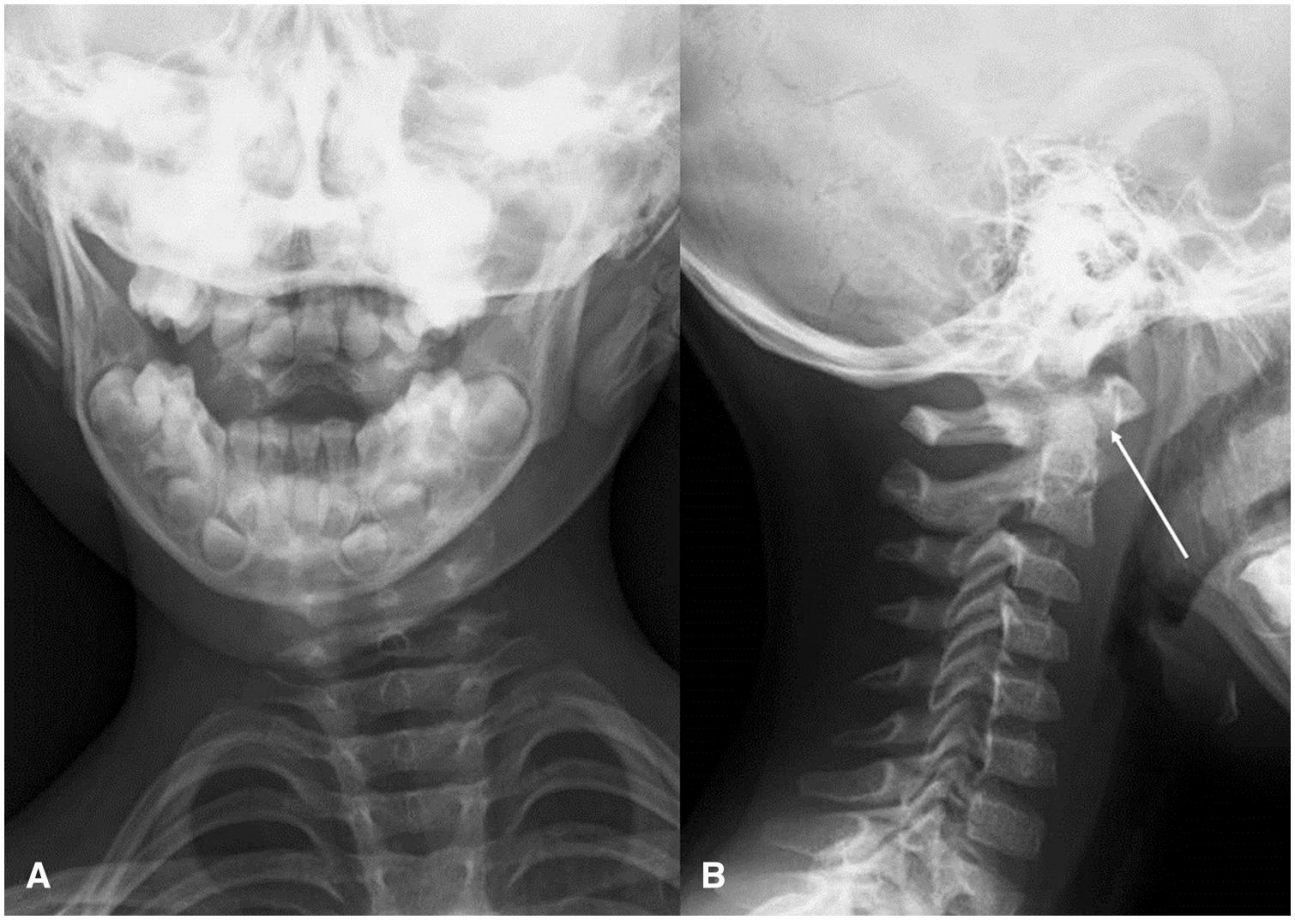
Routine plain cervical radiographs in 2 directions. (A) Anteroposterior view of the cervical spine showing head tilting. The atlantoaxial joint is difficult to evaluate in this image. (B) The lateral view. Atlanta-dental interval (arrow) was within the normal range at 4.4 mm.
